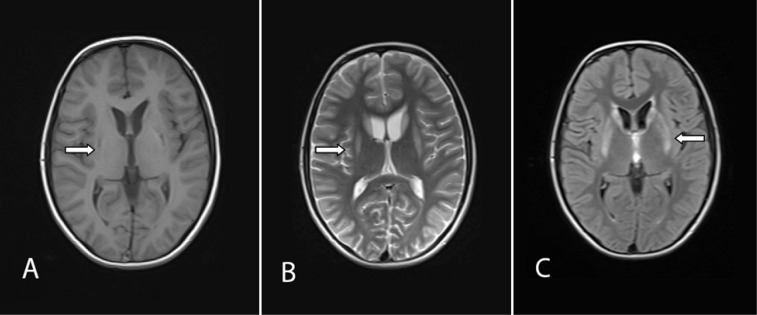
Brain Magnetic Resonance Imaging; Axial views. (A) T1- weighted sequence: decreasing signal intensity of bilateral putamen. (B) Hyper signal lesions of bilateral caudate and putamen nucleuses in T2 - weighted sequence. (C) Fluid Attenuated Inversion Recovery (flair) sequence. Bilateral involvement of putamen and head of caudate nucleus is seen
